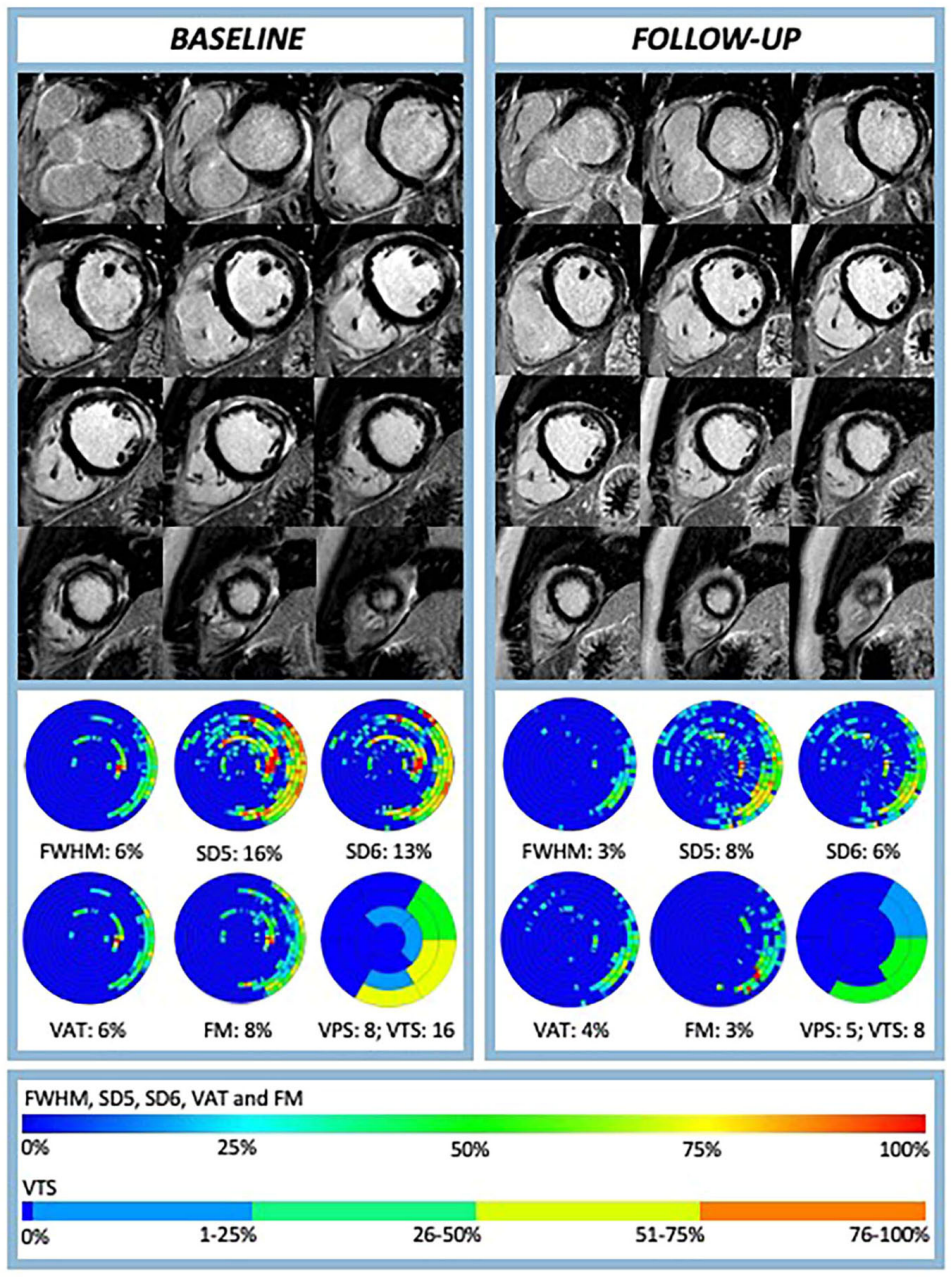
Example of LGE assessment using various quantitative techniques and visual scoring. Cardiovascular magnetic resonance images at baseline and 3-month follow-up of a 35-year-old male with acute myocarditis. Upper panels show LGE images in short-axis orientation, while lower panels present bulls-eye views with quantitative and visual scores. The color scale represents the LGE extent. Changes in LGE extent (percentage, %) from baseline to follow-up: FWHM 6% to 3%, SD5 16% to 8%, SD6 13% to 6%, VAT 6% to 4%, FM 8% to 3%. VPS decreased from 8 to 5, and VTS reduced from 16 to 8, corresponding a VCS of -3, indicating a 51–75% reduction in LGE
